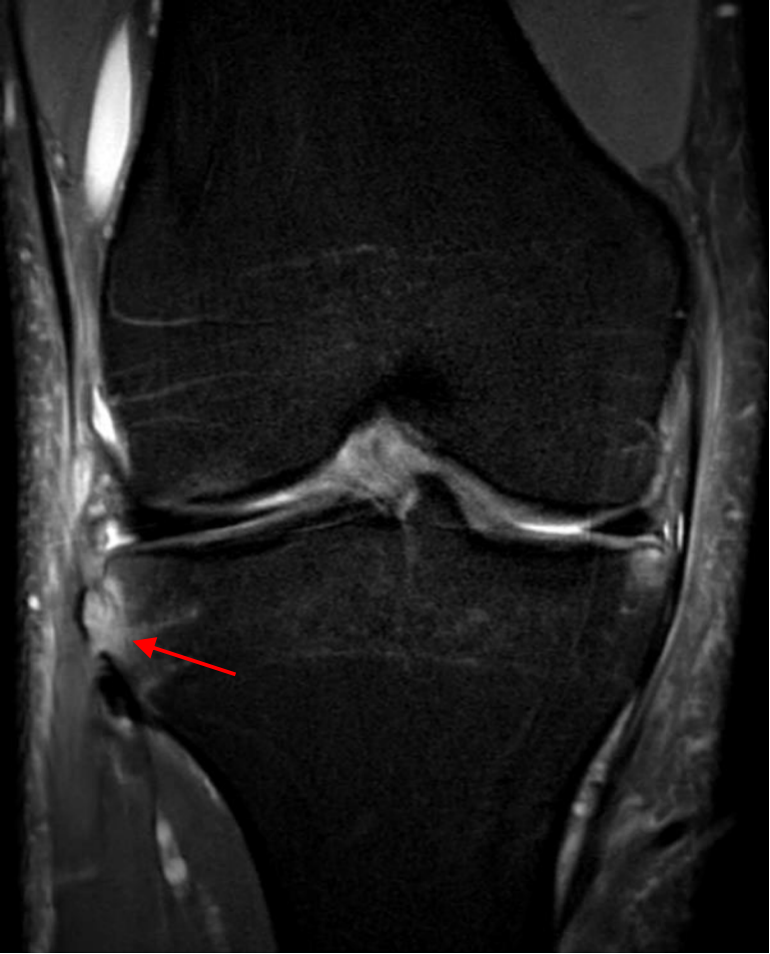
Segond fracture (arrow) on coronal proton density fat saturation magnetic resonance imaging (right knee)
